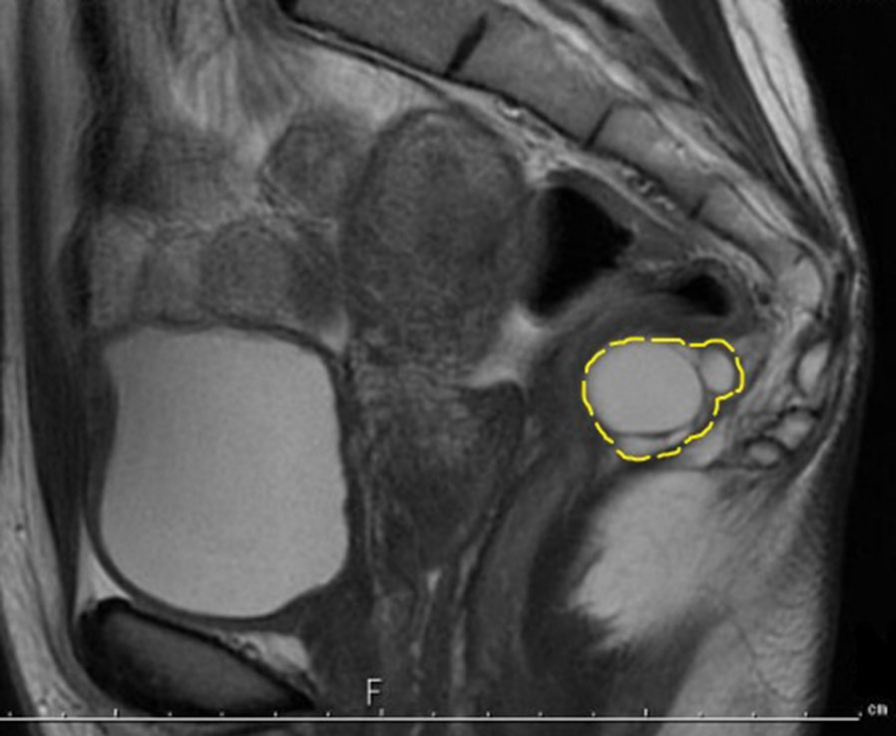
The tumor had a clear, smooth border and showed high signal intensity on T2-weighted images (dotted line). No fat was found in the tumor
